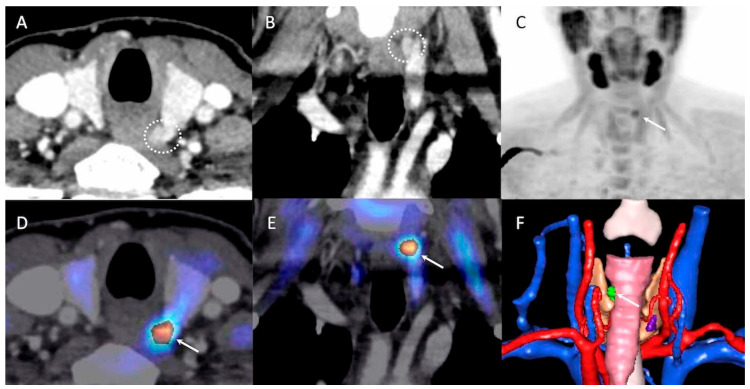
52-year-old woman with recurrent primary hyperparathyroidism (pHPT) and previous history of surgical ablation of left inferior parathyroid adenoma. First-line imaging was negative. Preoperative positron emission tomography/four-dimensional computed tomography (PET/4D-CT) showed increased focal ^18^F-FCH uptake (arrow) corresponding to 1 cm nodule located behind the superior pole of left thyroid lobe (left upper quadrant), with a typical contrast media enhancement (dotted circle). Parathyroid adenoma (0.1 g, 9 mm) was confirmed by pathology. (A,B): four-dimensional computed tomography 4D-CT, axial and coronal slices; (C): ^18^F-Fluorocholine positron emission tomography ^18^F-FCH PET, anterior maximum intensity projection (MIP); (D,E): PET/4D-CT, axial and coronal slices; (F): 3D-virtual neck exploration, posterior view; green: left upper parathyroid, rose: esophagus, orange: thyroid.
